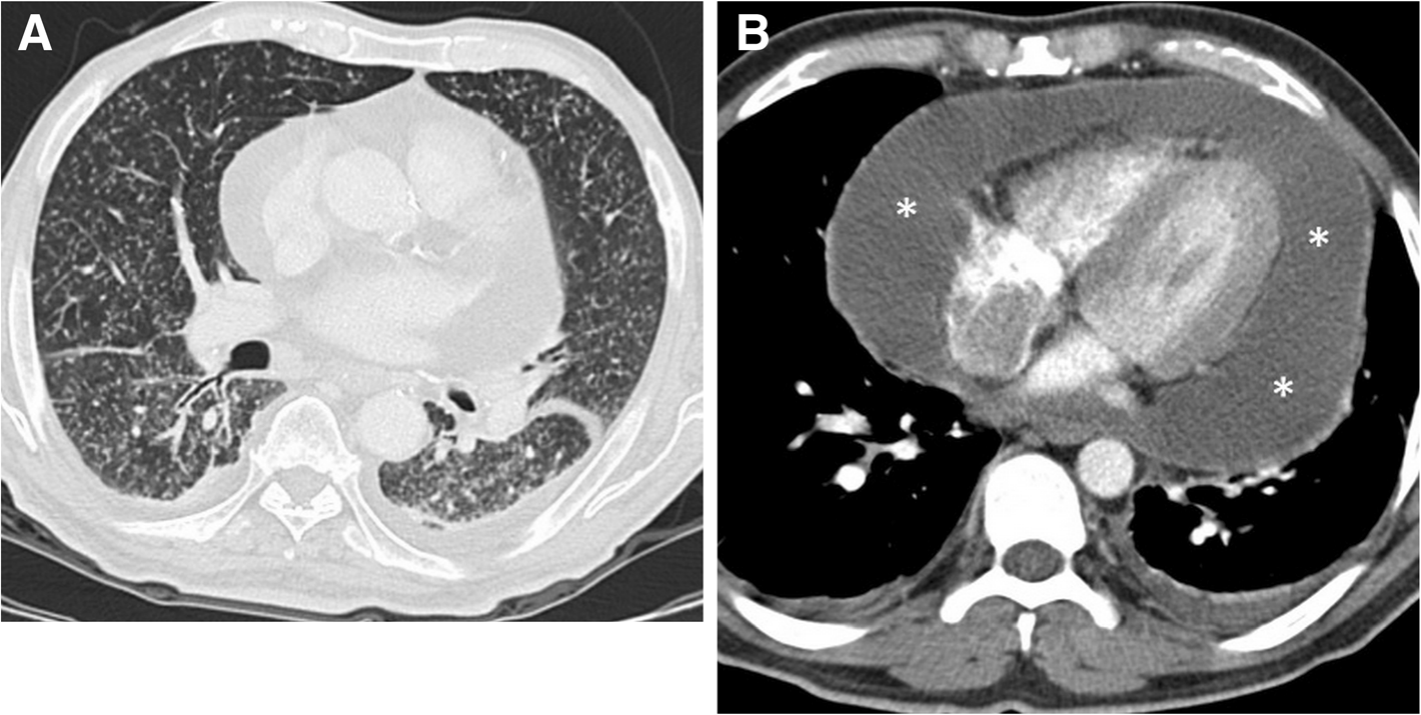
A 41-year-old man with newly diagnosed pulmonary tuberculosis. a Axial contrast-enhanced CT image at lung window reveals miliary pattern of pulmonary involvement. b Large amount of pericardial effusion (asterisks) is also noted. A culture test was positive for tuberculosis following the US-guided pericardiocentesis
